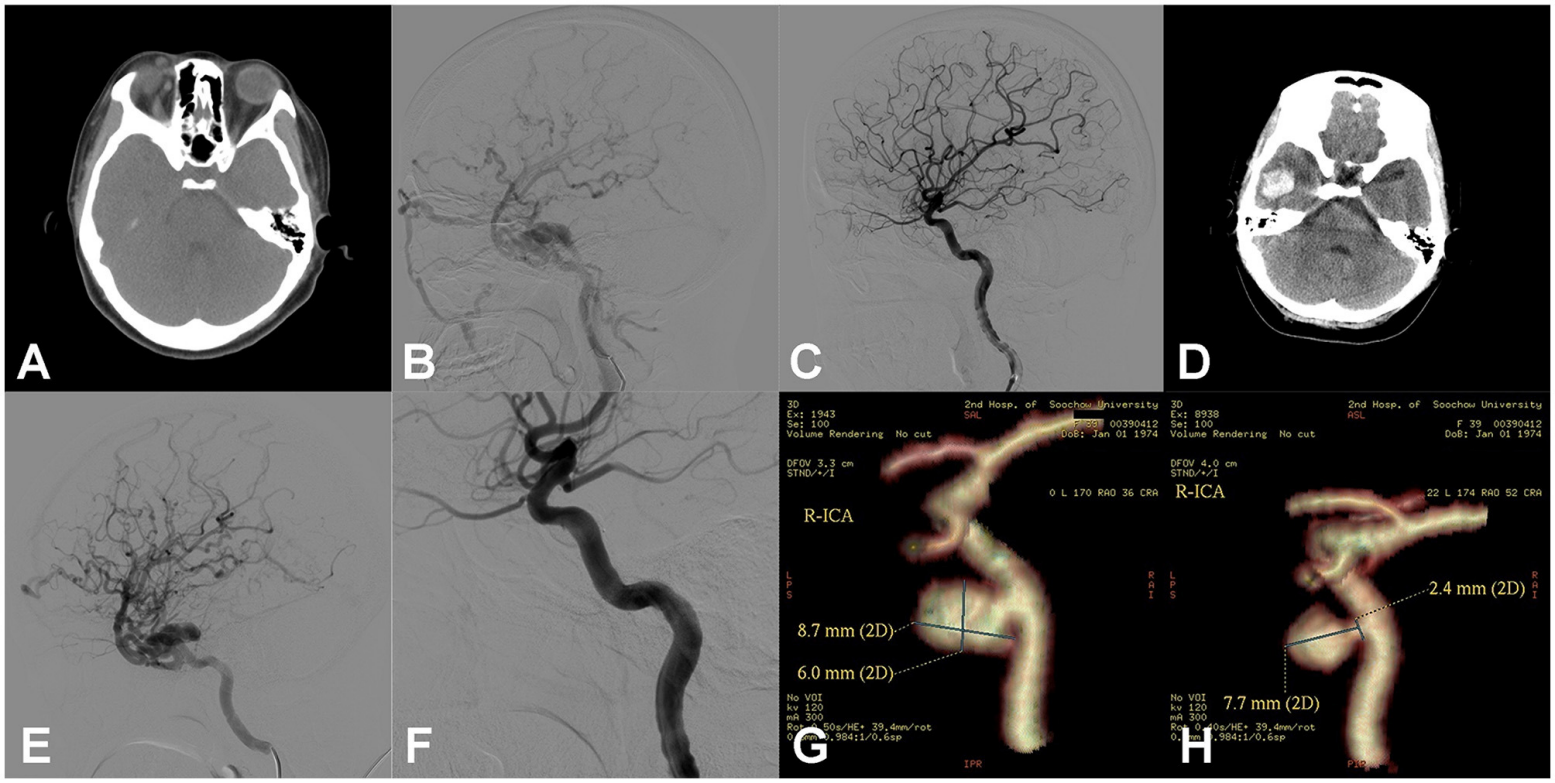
Right carotid cavernous fistula (CCF). An adult patient presented with right pulsing tinnitus after head trauma 1 month ago. Physical examination revealed a blowing murmur consistent with the heartbeat on the right temporal scalp, as well as a mild chemosis of the right side. (A) Head computed tomography (CT) showed the dilation of the right superior ophthalmic vein (SOV). (B) Digital subtraction angiography (DSA) of the right ICA showed the CCF with reflux drainage of cortical veins. (C) After dilation of one detachable balloon (Goldbal2), the pathological shunt disappeared and the cerebral blood flow recovered. The patient recovered well, and the symptoms of right chemosis and intracranial murmurs disappeared. (D) After 2 weeks, the patient presented to emergency with a sudden headache, and the head CT showed intracerebral hematoma of the right temporal lobe. The right temporal auscultation noted the recurrence of the blowing murmur. (E) Emergency DSA of the right ICA showed the recurrence of CCF and the reflux drainage of the cortical veins aggravated compared to previous images besides the undevelopment of SOV. (F) Another detachable balloon (Goldbal2) was introduced in the cavernous sinus, and the pathological shunt disappeared angiographically. The patient recovered well after the endovascular procedure with no neurological deficits. (G) After 3 months, CT angiography (CTA) showed the balloon had deflated and there was a consequent pseudoaneurysm in the cavernous sinus. The patient hesitated to receive another endovascular treatment. (H) In the 3-year follow-up, CTA showed the pseudoaneurysm was still present but smaller than before.
